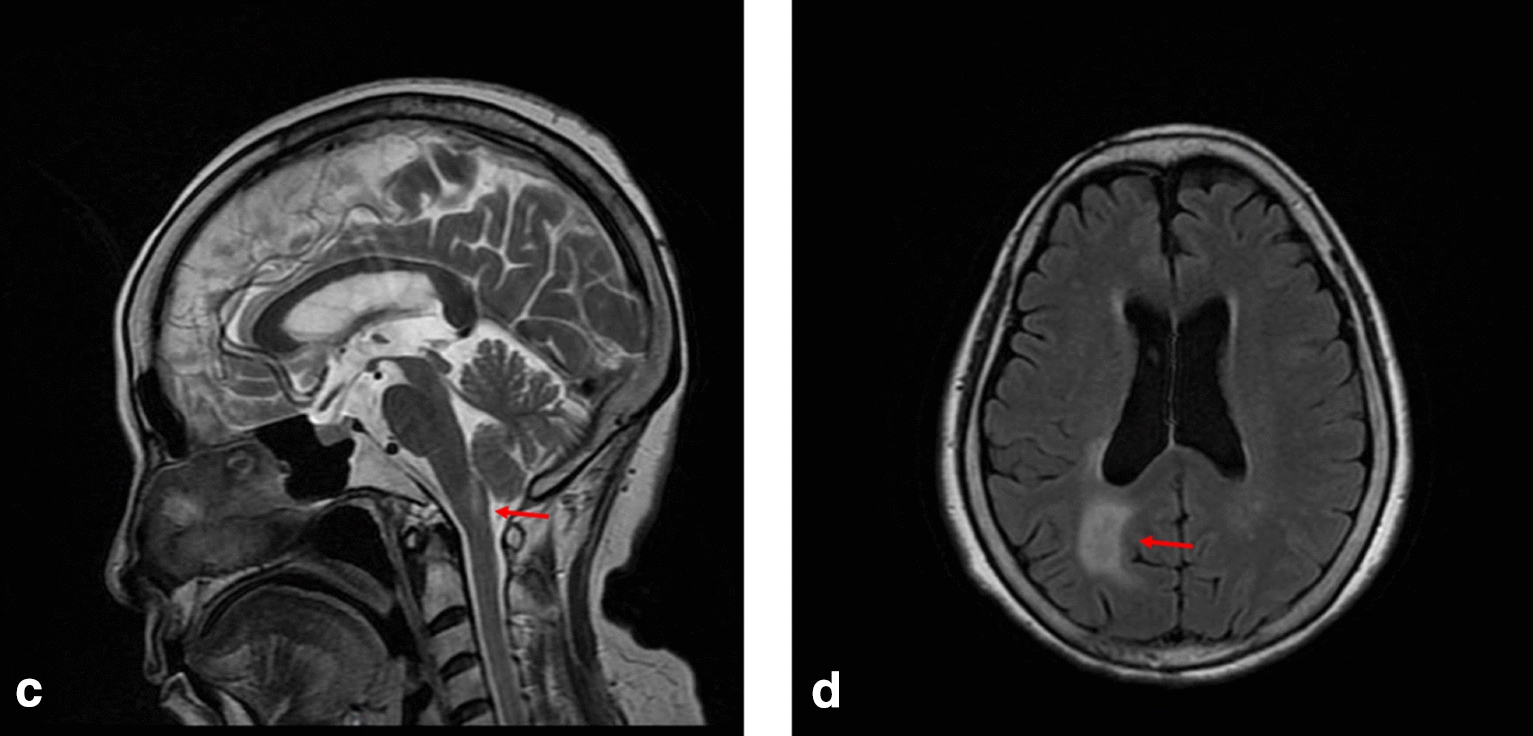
MRI T2 sequence of case 2 showing hyperintense in medulla oblongata (highlighted by arrows) in the c sagittal planes, and hyperintense in the right paraventricular and left occipital lobe in the d transverse planes
